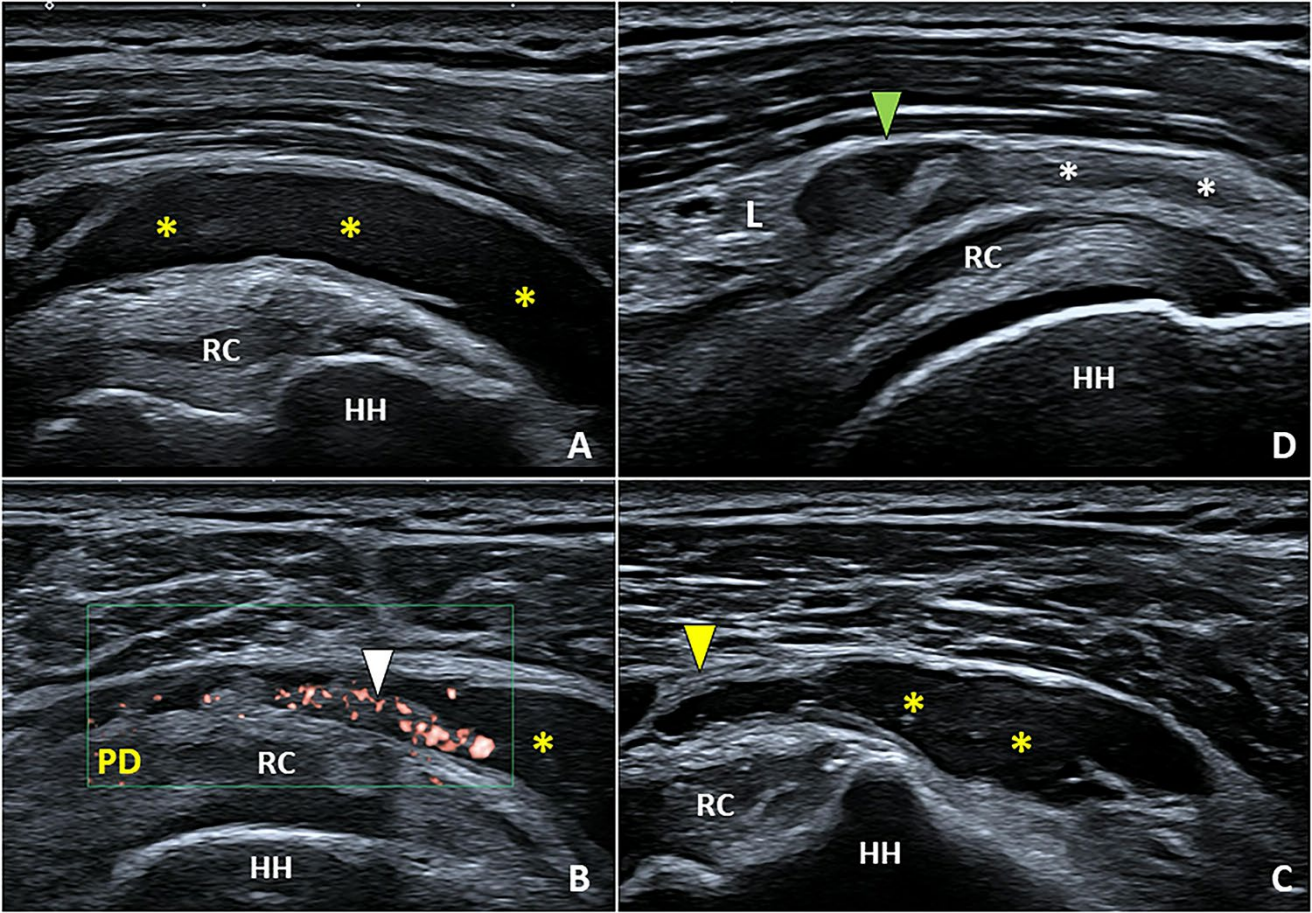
In the acute phase, the effusion *(yellow asterisks)* is the most common sonographic finding defining exudative bursitis (A). Progressively, synovial hypertrophy (B) with hypervascularization *(white arrowhead)* and fibrotic septae *(yellow arrowhead)* can develop within the bursal cavity (C). Adhesive bursopathy (D) shows a well-defined, hypoechoic, nodular thickening *(green arrowhead)* of the synovial tissue *(white asterisk)* close to the overlying hyperechoic coracoacromial ligament *(L)*. *HH* humeral head, *RC* rotator cuff, *PD* power Doppler
